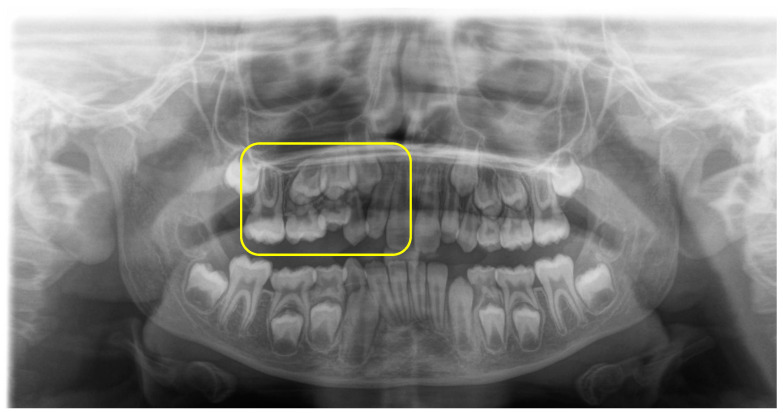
Orthopantomography (OPT)to determine the permanent successors’ status and the main molars’ position.
